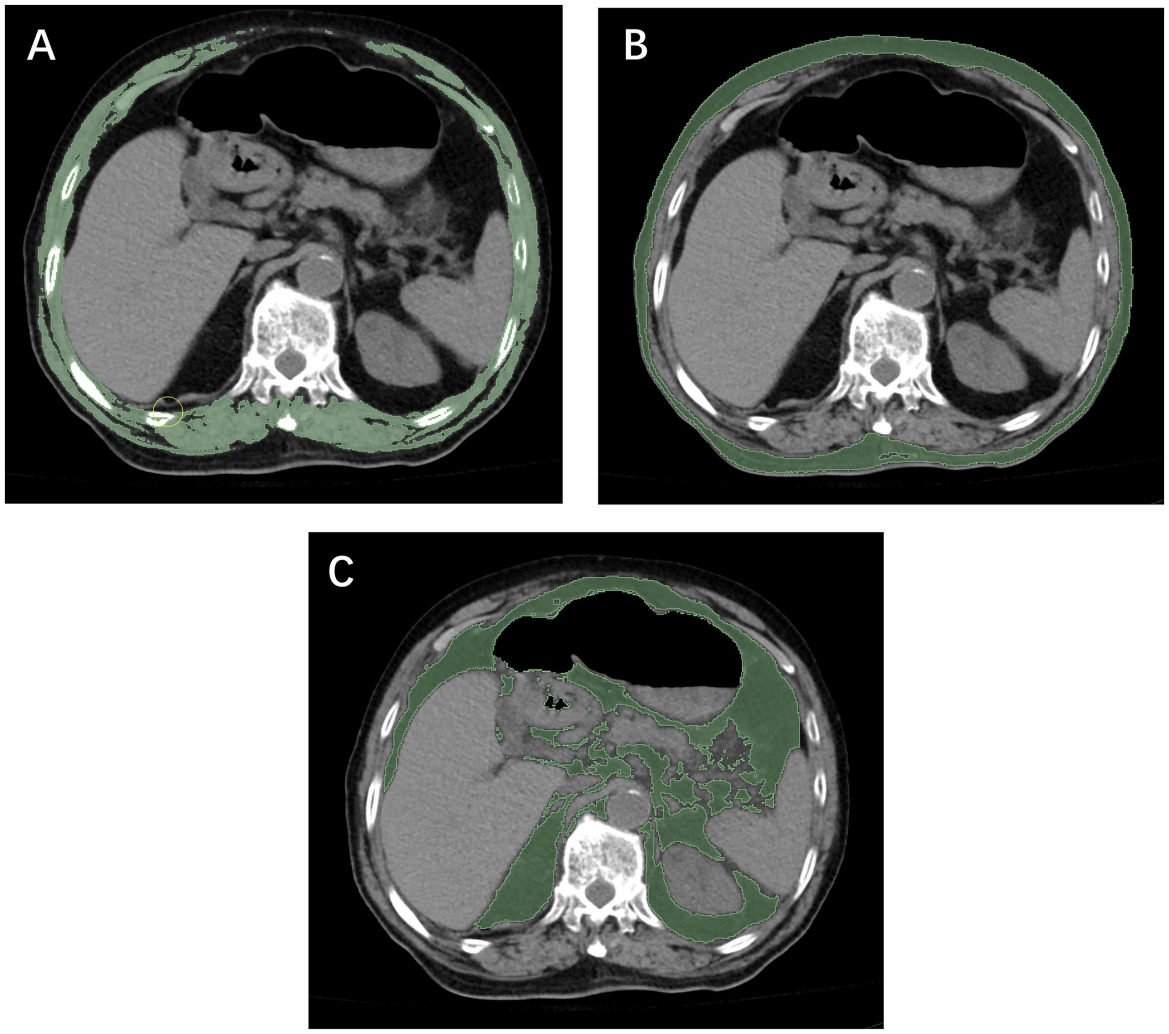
Measurement of the skeletal muscle index (A), subcutaneous fat index (B), and visceral fat index (C) using computed tomography at the T12 level.
